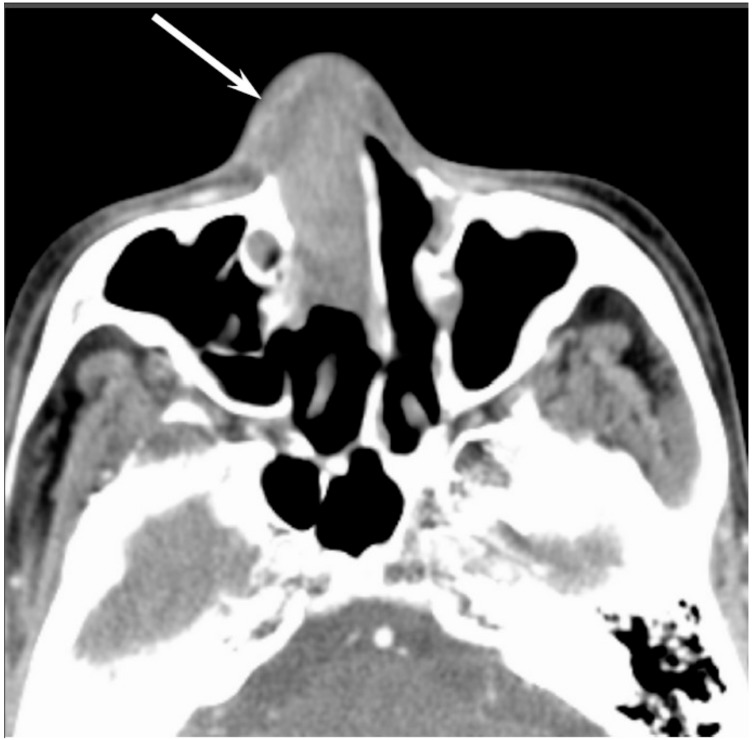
Sinonasal undifferentiated carcinoma of the right nasal cavity. Contrast-enhanced CT image showing an ill-demarcated, heterogeneously enhanced lesion (arrow).
